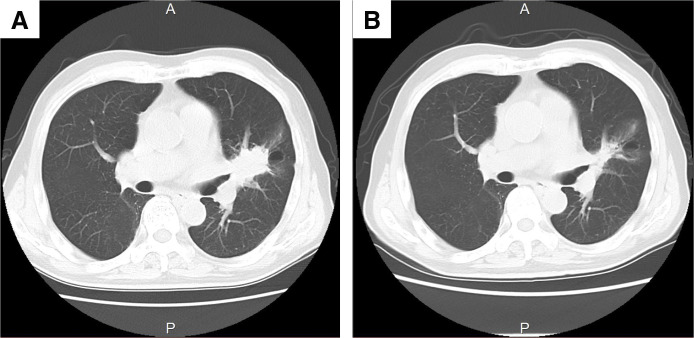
CT images obtained (A) before and (B) after neoadjuvant chemotherapy. The tumor decreased in size following chemotherapy, and no shadows suggestive of ILD were observed before or after treatment.
